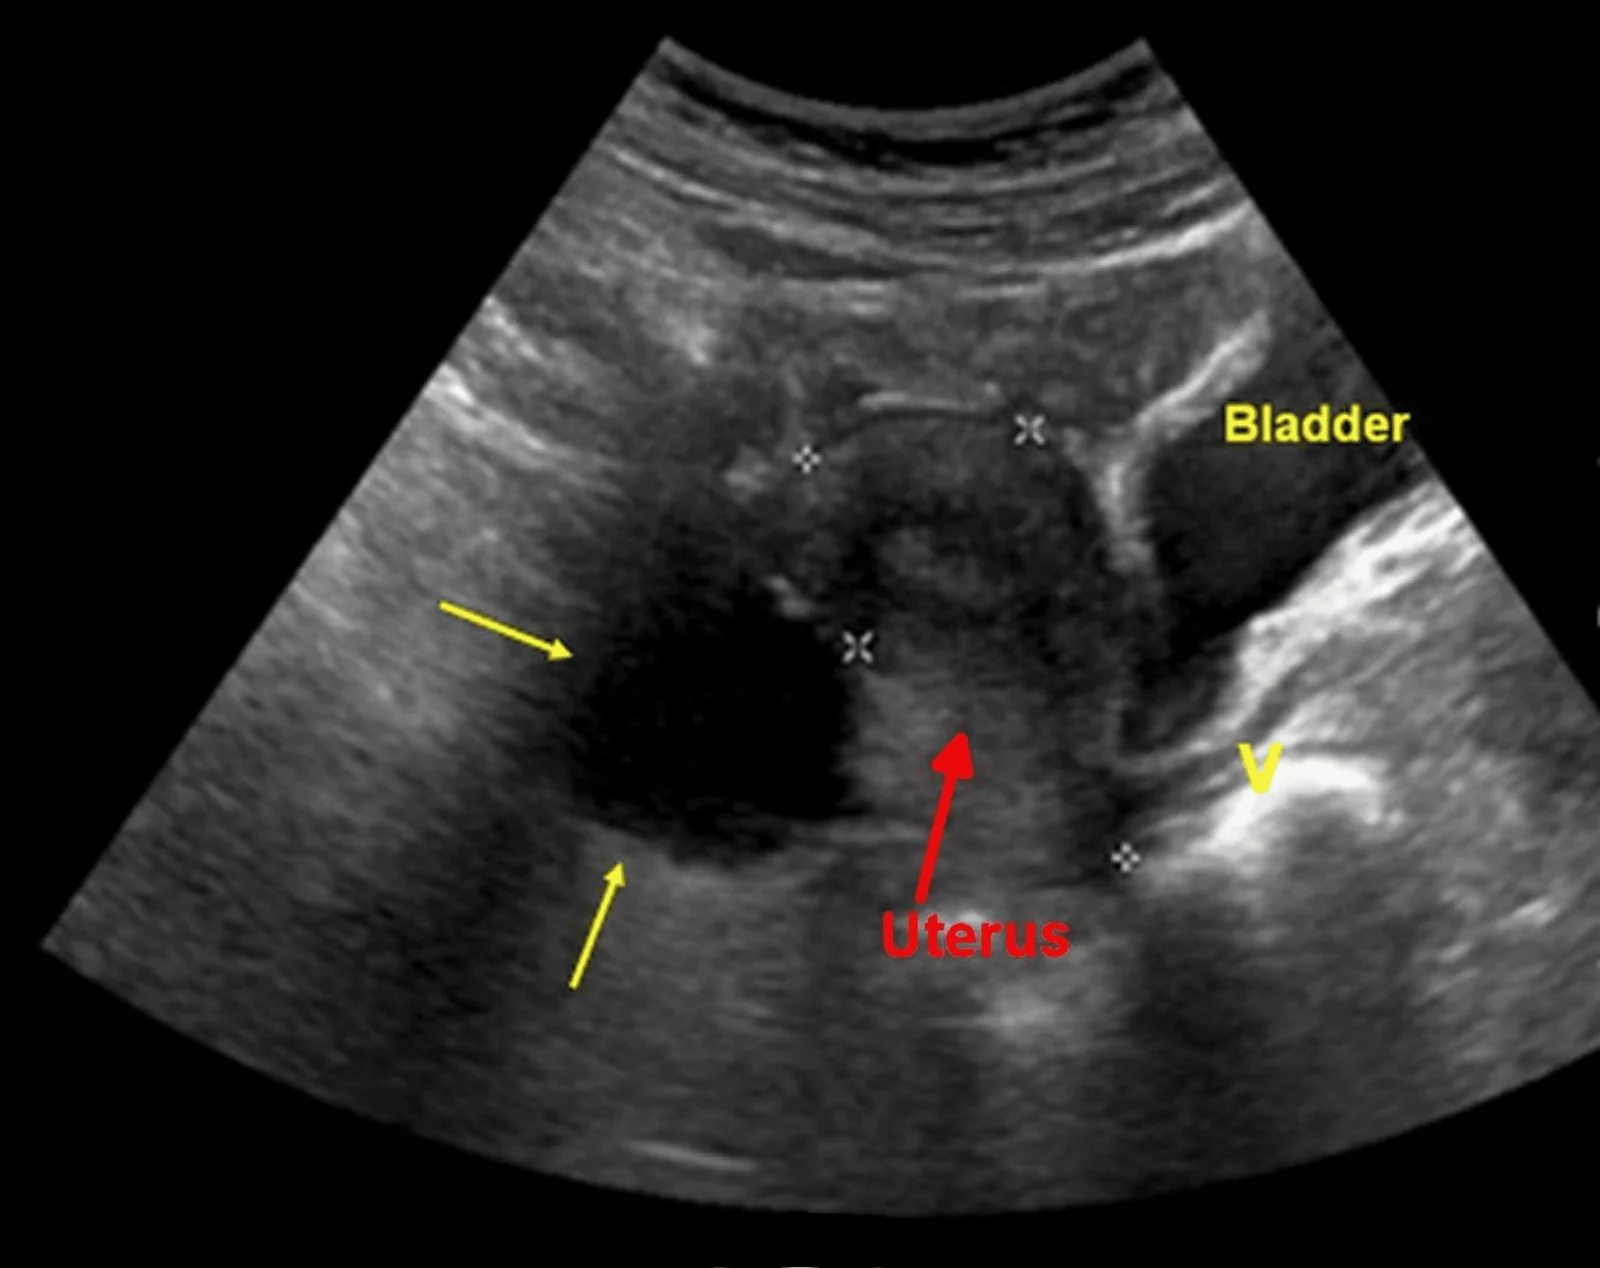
Initial transabdominal pelvic ultrasound The uterus, indicated by the cursors, measures 5.59 × 3.0 cm (red arrow). The yellow arrows indicate a clear cystic structure posterior to the uterus, with associated acoustic shadowing. No free fluid or evidence of pregnancy is identified.
